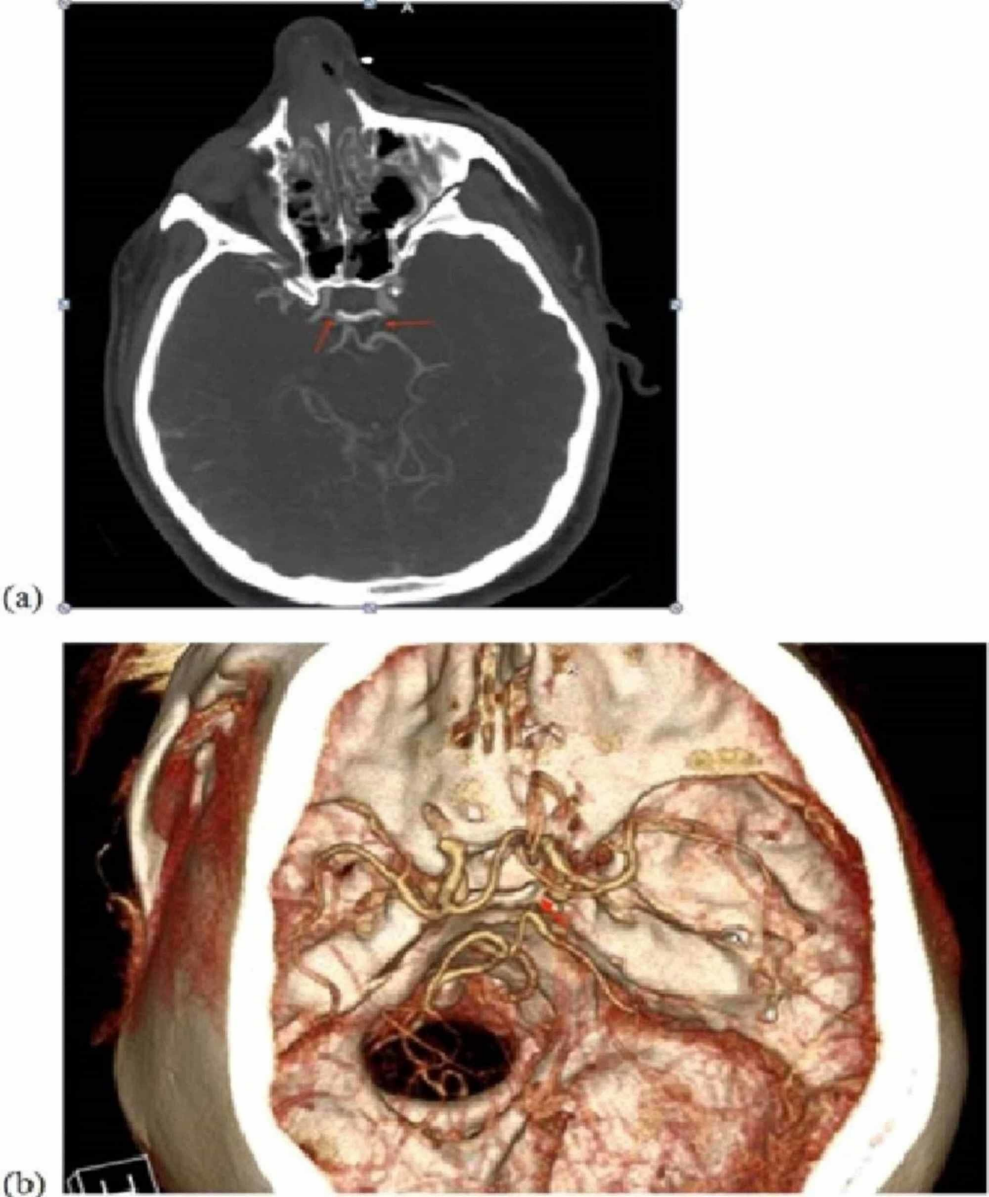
CT angiography of the brain: (a) axial cut with arrows indicating the right and left posterior communicating arteries, respectively. (b) CT angiography reconstruction demonstrating a patent right posterior cerebral artery.
